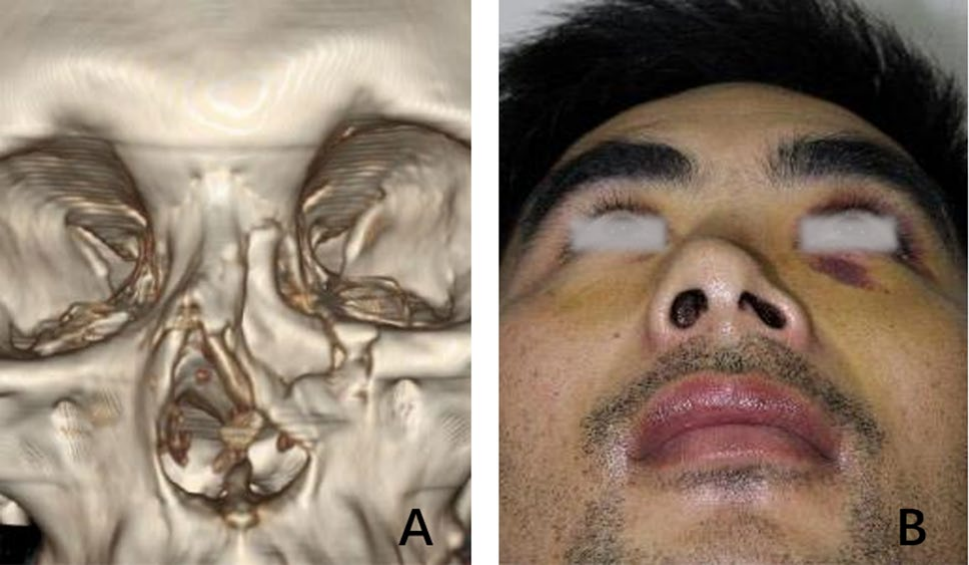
Comminuted type II fracture. Three-dimensional and horizontal computed tomography images reveal the collapse of the left two-thirds of the nasal vault and middle turbinate (A). An obvious asymmetry of the nostrils was noticed (B)
